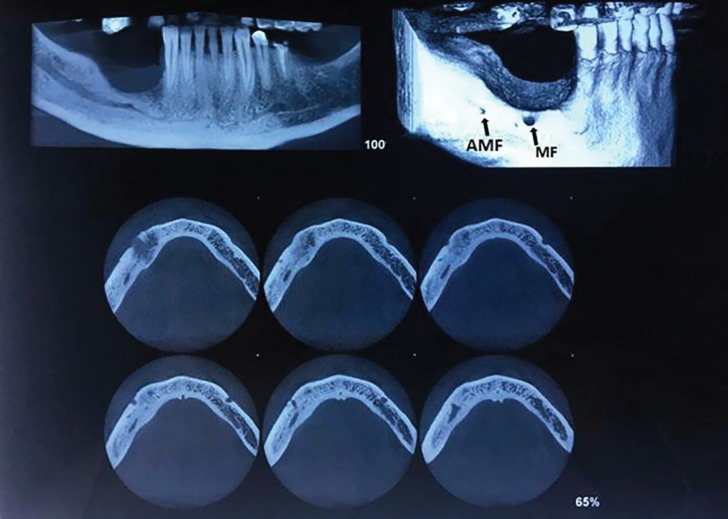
The diagnosis of AMF posterior to main mental foramen confirmed by CBCT
